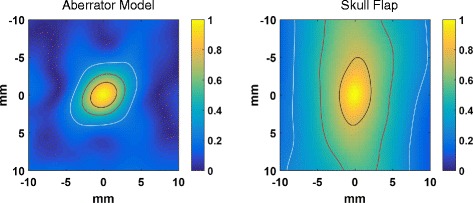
Autocorrelation of path lengths. The normalized autocorrelation of the phase lengths for the aberrator model (*left*) and skull flap (*right*) along the axis of the ultrasound propagation. *Contour lines* are displayed for values of 25 % (*white*), 50 % (*red*), and 75 % (*black*) of the maximum correlation
